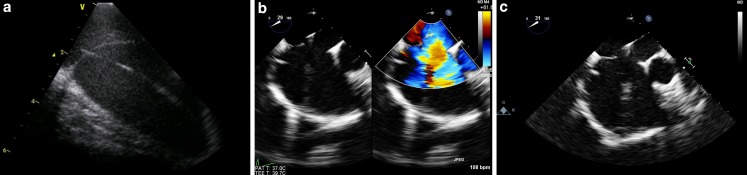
Intracardiac echocardiography compared with microtransoesophageal echocardiographic images. Intraprocedural images during atrial septum defect (ASD) II closure in an adult patient. a Intracardiac echocardiography (ICE) showing the intra-atrial septum and the catheter. b Microtransoesophageal echocardiography (TEE) colour compare mode showing the ASD (left) and the flow through the ASD (right) in a short axis at the base view. c MicroTEE short axis at the base view, showing a much wider field of view (visibility of the aorta) compared with ICE in (a)
